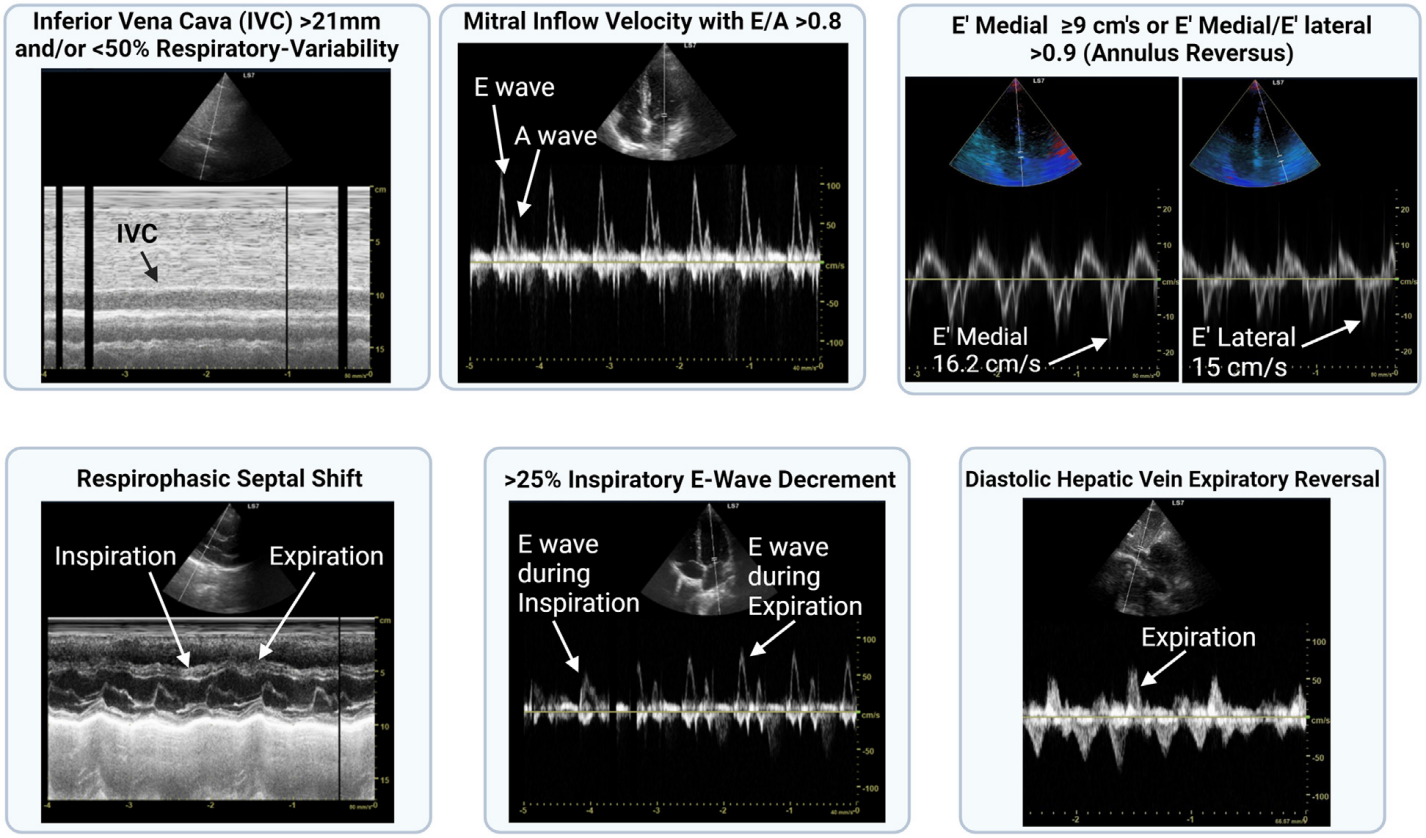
Echocardiographic Findings of Pericardial Constriction Various signs of constriction observed during the study.
